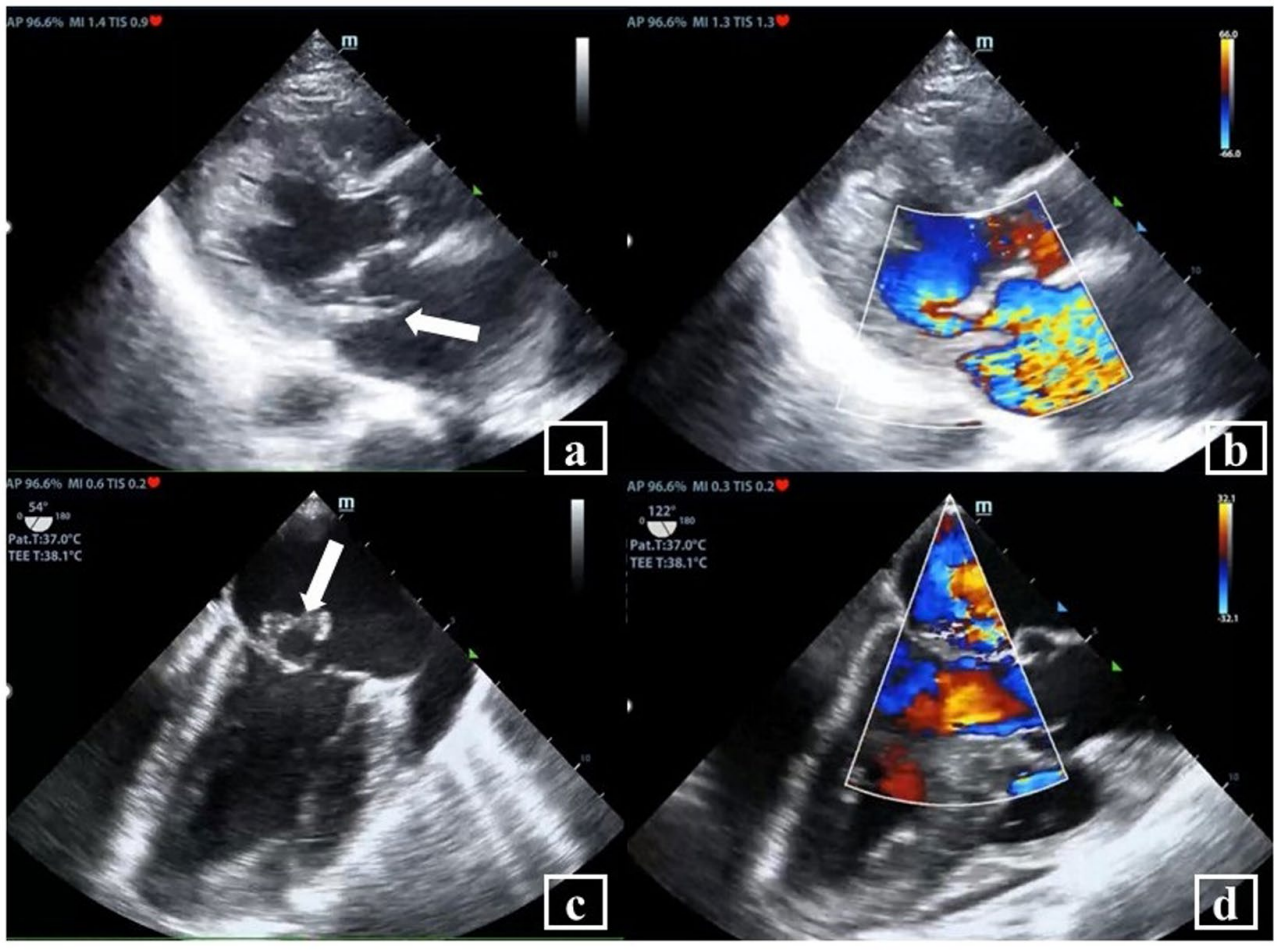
Transthoracic echocardiography (TTE) and transesophageal echocardiography (TEE). (a) TTE showed posterior mitral valve tendon cord rupture and posterior mitral valve prolapse (MVP) (arrow); (b) severe mitral regurgitation (MR) was observed on Doppler color view (TTE); (c) TEE showed a vegetation on the mitral valve and the vegetation was about 13 × 4.0 mm (arrow); (d) severe eccentric regurgitation signal of the mitral regurgitation (MR) was observed on Doppler color view (TEE).
